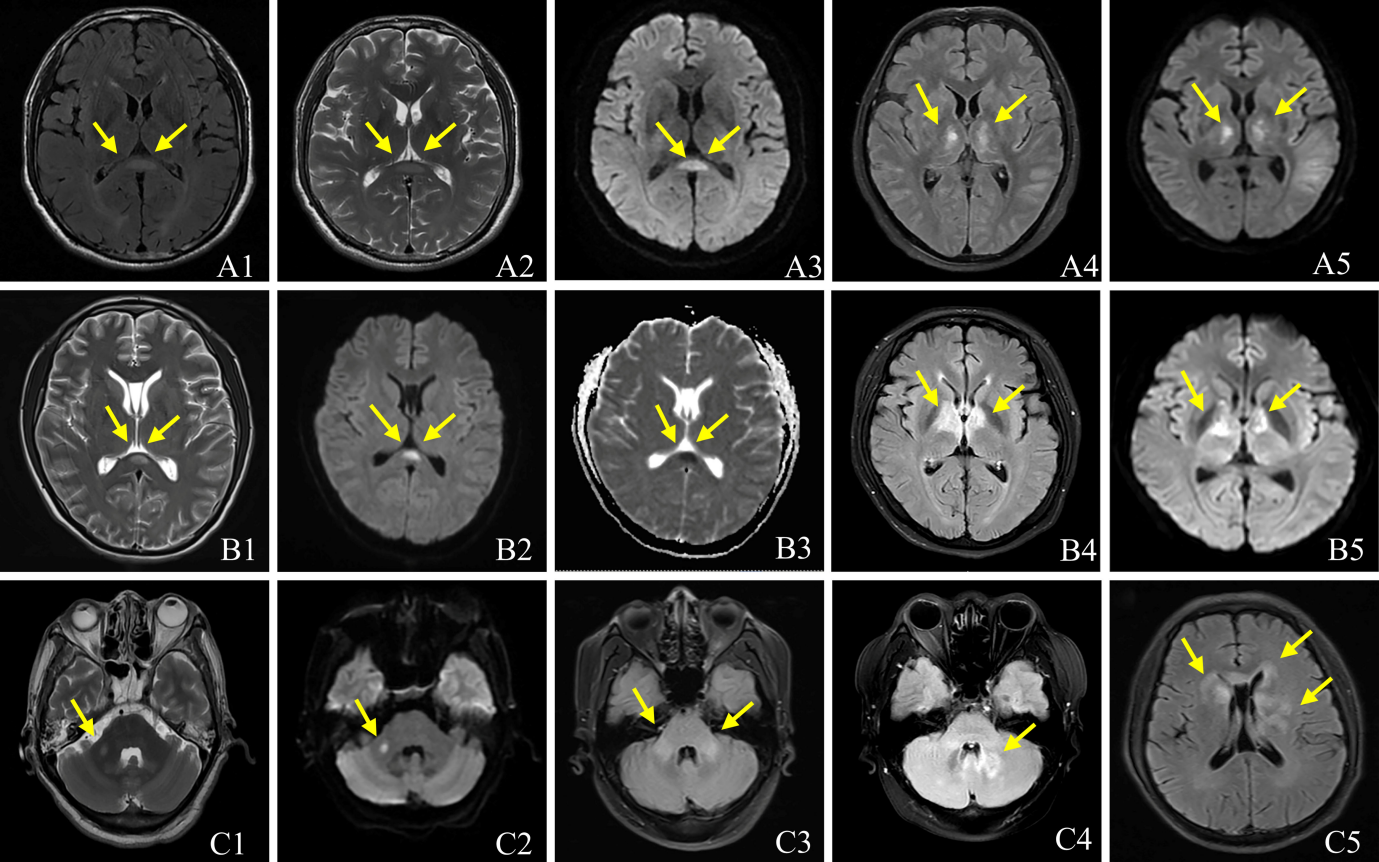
Brain MRI characteristics of patients with Anti-GFAP antibodies. The imaging looks like a reversible splenial lesion (A1-A3, B1-B3). T2 FLAIR (A4, B4) and Diffusion Weighted Imaging (A5, B5) show lesions in bilateral thalamus, T2-weighted (C1) image and FLAIR (C2, C3) show lesions in brachium pontis; T2 FLAIR shows lesions in cerebellum (C4), basal ganglia (C5) and paraventricular (C5).
